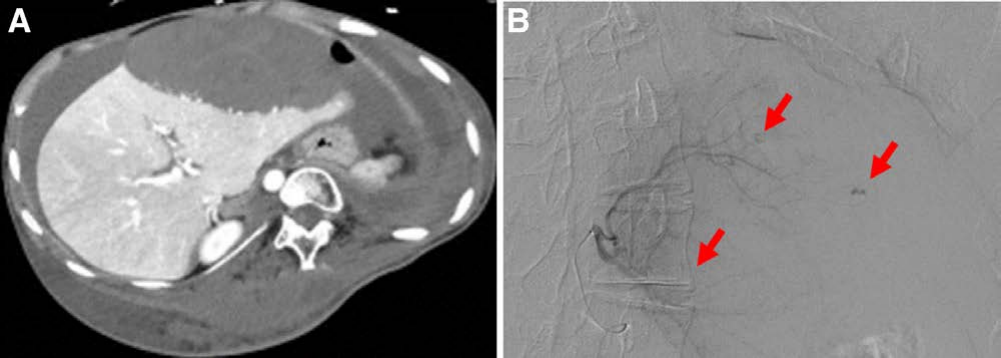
Computed tomography shows a massive hepatic subcapsular hemorrhage (A). Hepatic arteriography revealed a number of hemorrhage points (red arrow). However, no obvious hepatic arterial malformations were found (B).
